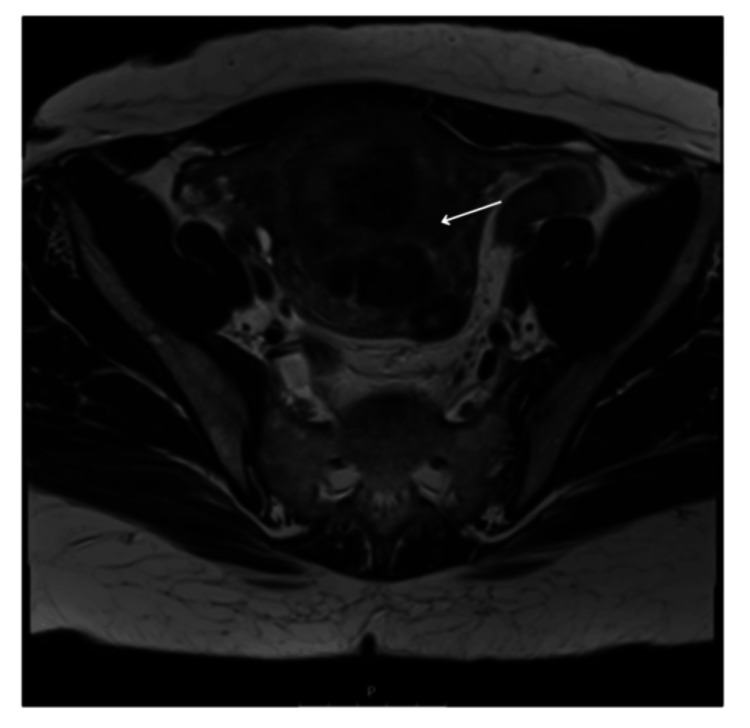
Axial large field of view T2 weighted MRI image of the pelvis showing uterine fibroids (arrow), pre-embolisation
